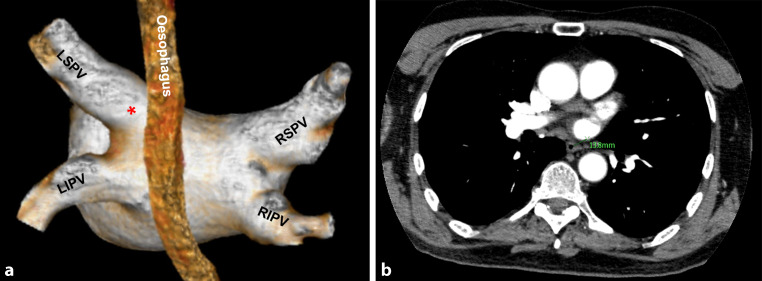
a Shortest oesophagus to pulmonary vein distance measurement when we first looked at the CT images. The origin of the os, identified as the indentation in the posterior wall, caused by the angulation of the pulmonary vein compared to the atrial wall. The angulation between the pulmonary vein and the atrium can be well distinguished in this 3D view. The distance from the identified angulation (*) to the oesophagus in the axial plane represents the shortest oesophagus to pulmonary vein distance. *LSPV* left superior pulmonary vein, *RSPV* right superior pulmonary vein, *LIPV* left inferior pulmonary vein, *RIPV* right inferior pulmonary vein. b This distance was measured
